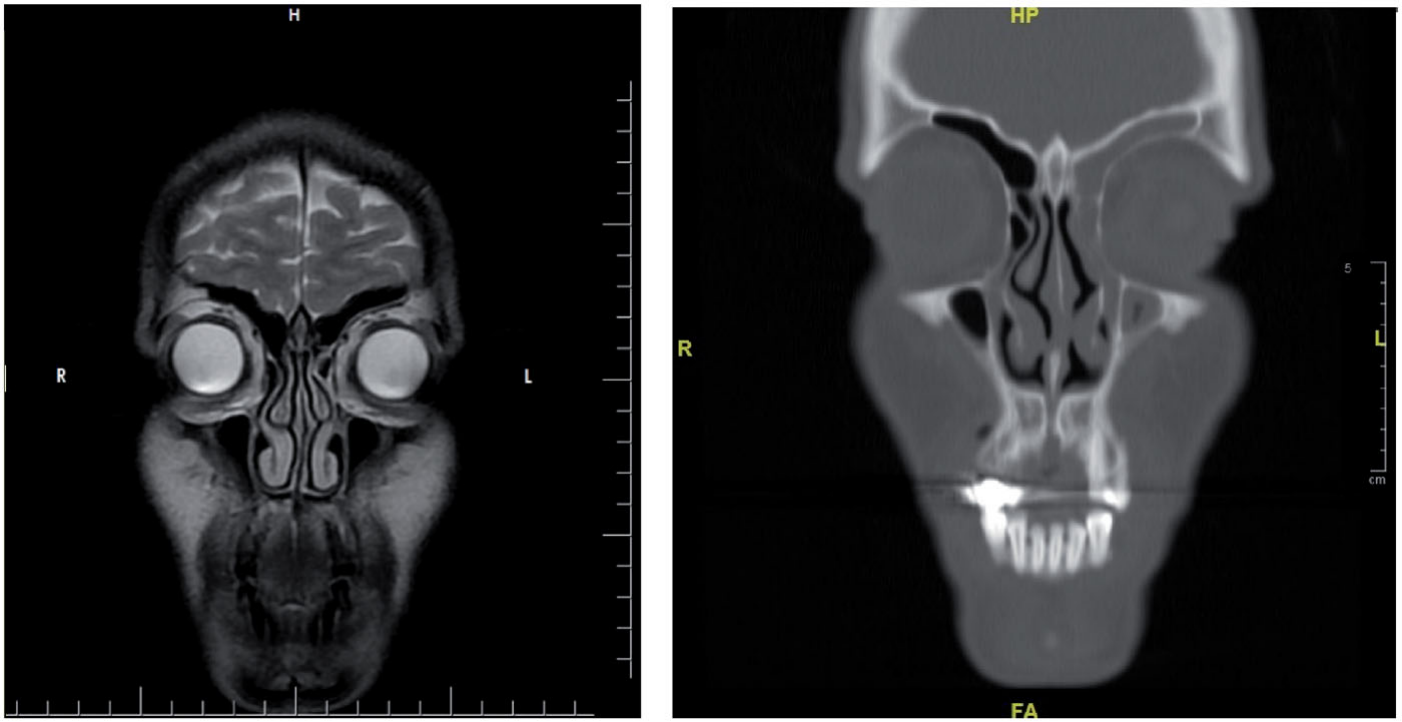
The comparison of the preoperative MR images (left) and the postoperative CT images (right). In the tomography of the patient, the opacification and atelectasis of the left maxillary, the left frontal and the left ethmoid sinuses are seen together in the coronal section. Also, the lateral displacement of the middle concha can be noted in the postoperative CT image.
